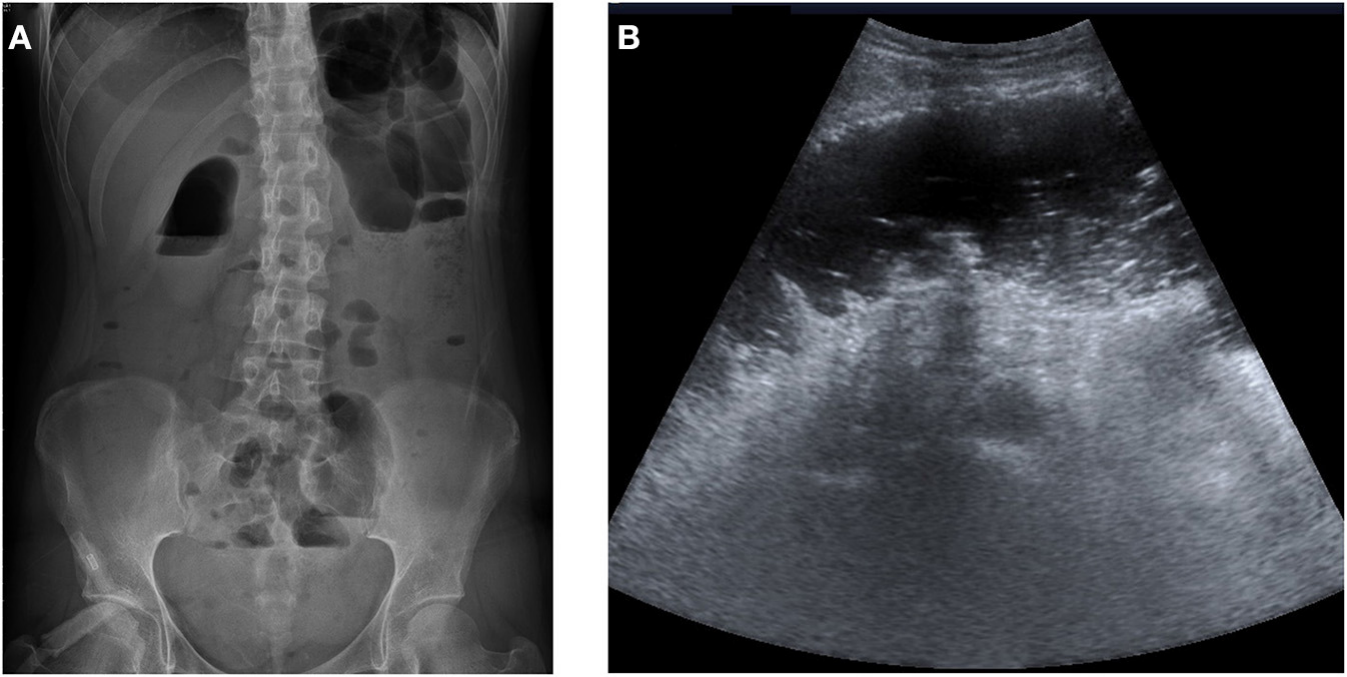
(A) The initial abdominal plain film showed non-specific bowel gas distribution without abnormal calcification or free air. (B) Ultrasonography of the abdomen revealed colon dilatation with air-fluid levels and ascites.
